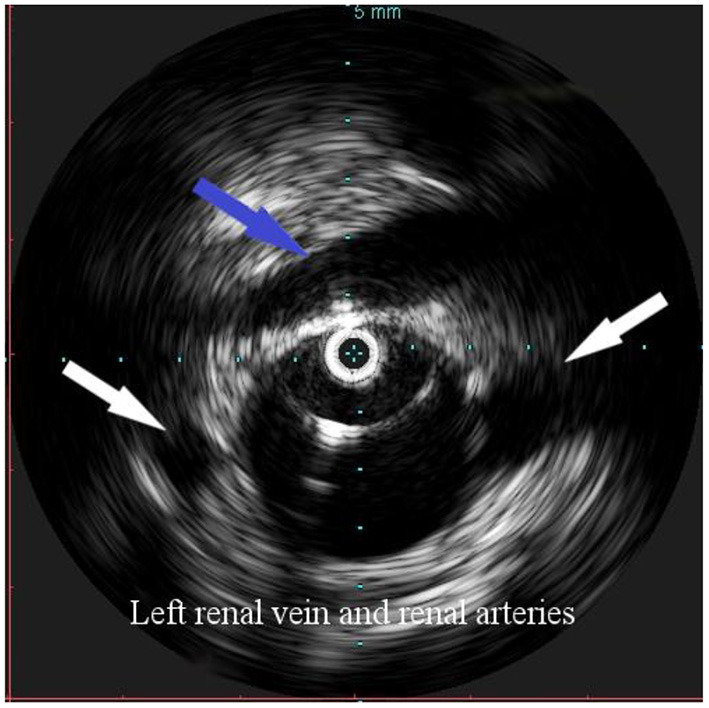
Intravascular ultrasound (IVUS) identification of the ostia of renal arteries with the left one being lower (white arrows), in order to define the proximal landing zone of the graft. The left renal vein can be seen crossing the anterior aortic wall (arrow).
